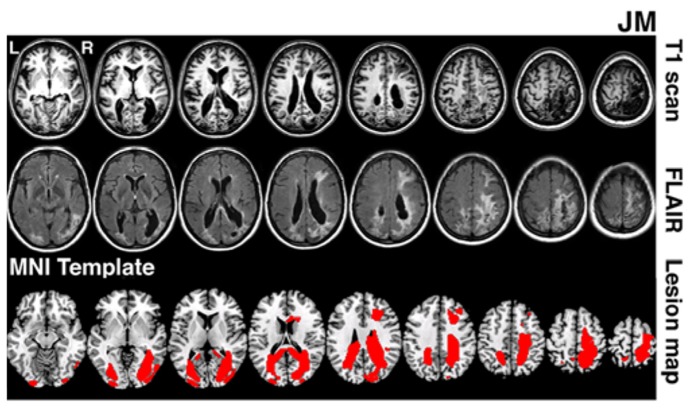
Lesion reconstruction for patient JM. The figure depicts T1 and T2 FLAIR structural scans (3T Philips Achieva MRI system with 8-channel phased array SENSE head coil). The bottom panel represents patient’s lesion map reconstructed based on outlier detection method combined with fuzzy clustering algorithm (see Seghier et al., 2008; Chechlacz et al., 2012). The lesion map is presented as an overlay on a standard T1 multi-slice template in MRIcron (Chris Rorden, Georgia Tech, Atlanta, GA, USA). L = Left and R = Right hemisphere.
